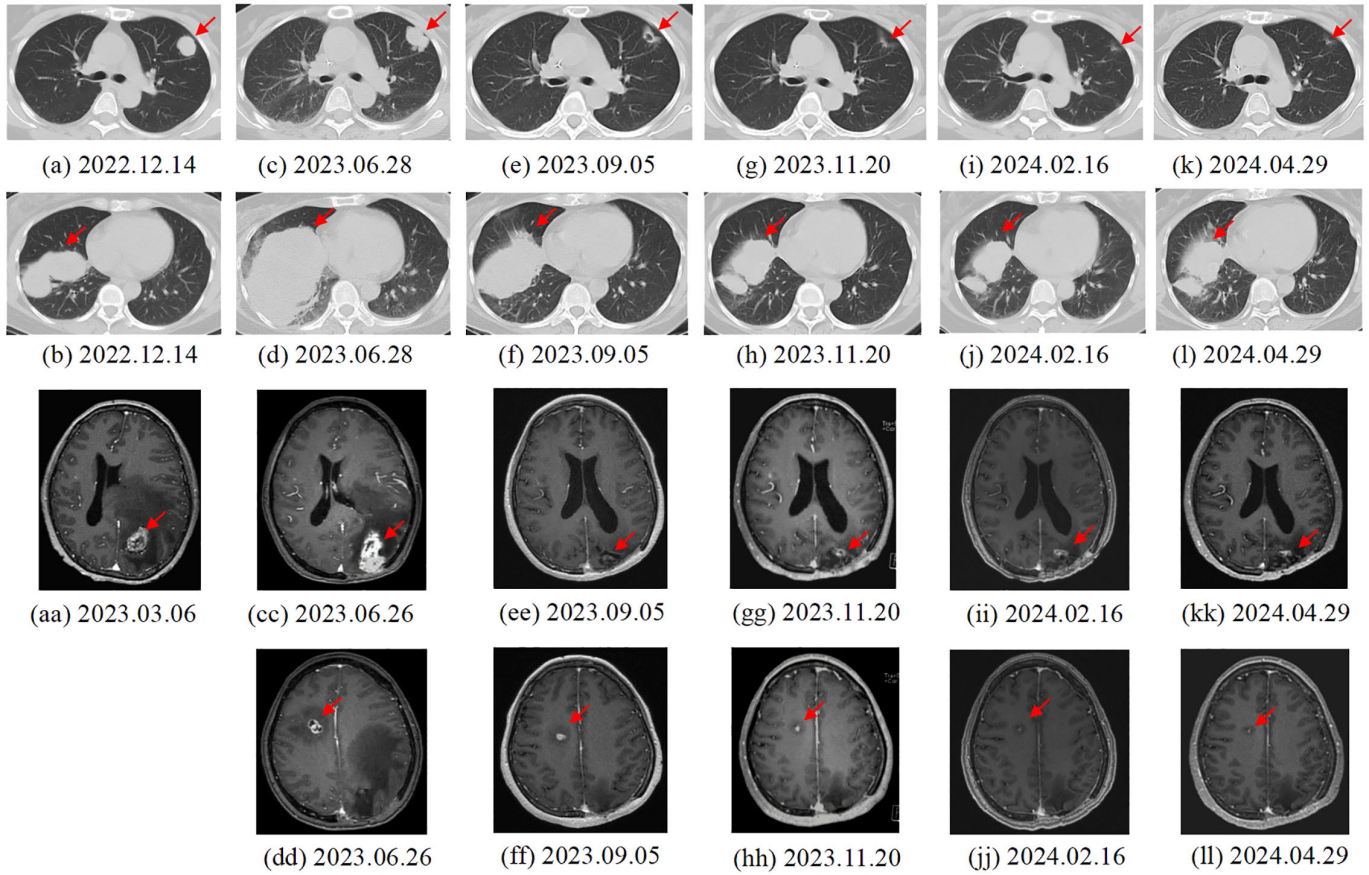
Changes in lung (a-l) and brain (aa-ll) metastases of the patient. (A, B) 2022.12.14. First recurrence: Results of chest CT scan. The largest tumor was 6.0 × 4.0 cm in size. (C, D) 2023.6.28. Third recurrence: Results of chest CT scan. The largest tumor was 10.6 × 6.9 cm in size. (E, F) 2023.9.05. After two cycles of cadonilimab: Results of chest CT scan. The largest tumor was 8.0 × 5.0 cm in size. (G, H) 2023.11.20. After five cycles of cadonilimab: Results of chest CT scan. (I, J) 2024.02.16. maintenance treatment of cadonilimab: Results of chest CT scan. The largest tumor was 5.8 × 2.7 cm in size. (K, L) 2024.04.29. maintenance treatment of cadonilimab: Results of chest CT scan. The largest tumor was 5.2 × 2.6 cm in size. (aa) 2023.3.06. Second recurrence: MRI and CT of the brain showed a single 3.6 × 2.5 cm metastasis within the left supratentorial lobe. (CC, DD) 2023.6.26. Third recurrence: Multiple brain metastases in the parenchyma on both sides. (EE, FF) 2023.9.05. After two cycles of cadonilimab: Results of brain MRI scan. The interval shank with largest tumor was 1.5× 0.8 cm in size (2e). (GG, HH) 2023.11.20. After five cycles of cadonilimab: Results of brain MRI scan. The interval shank with largest tumor was 1.1×0. 8 cm in size (2f). (II, JJ) 2024.02.16. maintenance treatment of cadonilimab: Results of brain MRI scan. The interval shank with largest tumor was 0.9×0. 7 cm in size. (KK, LL) 2024.04.29. maintenance treatment of cadonilimab: Results of brain MRI scan. The interval shank with largest tumor was 0.9×0. 7 cm in size.
